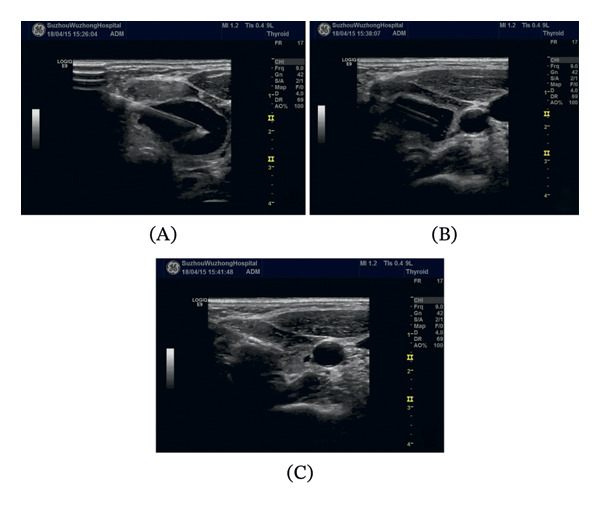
Ultrasound images from a 43‐year‐old woman with a predominantly cystic thyroid nodule before percutaneous lauromacrogol injection. (A) Indwelling needle puncture. (B) After removing the needle core. (C) Lauromacrogol irrigation.
